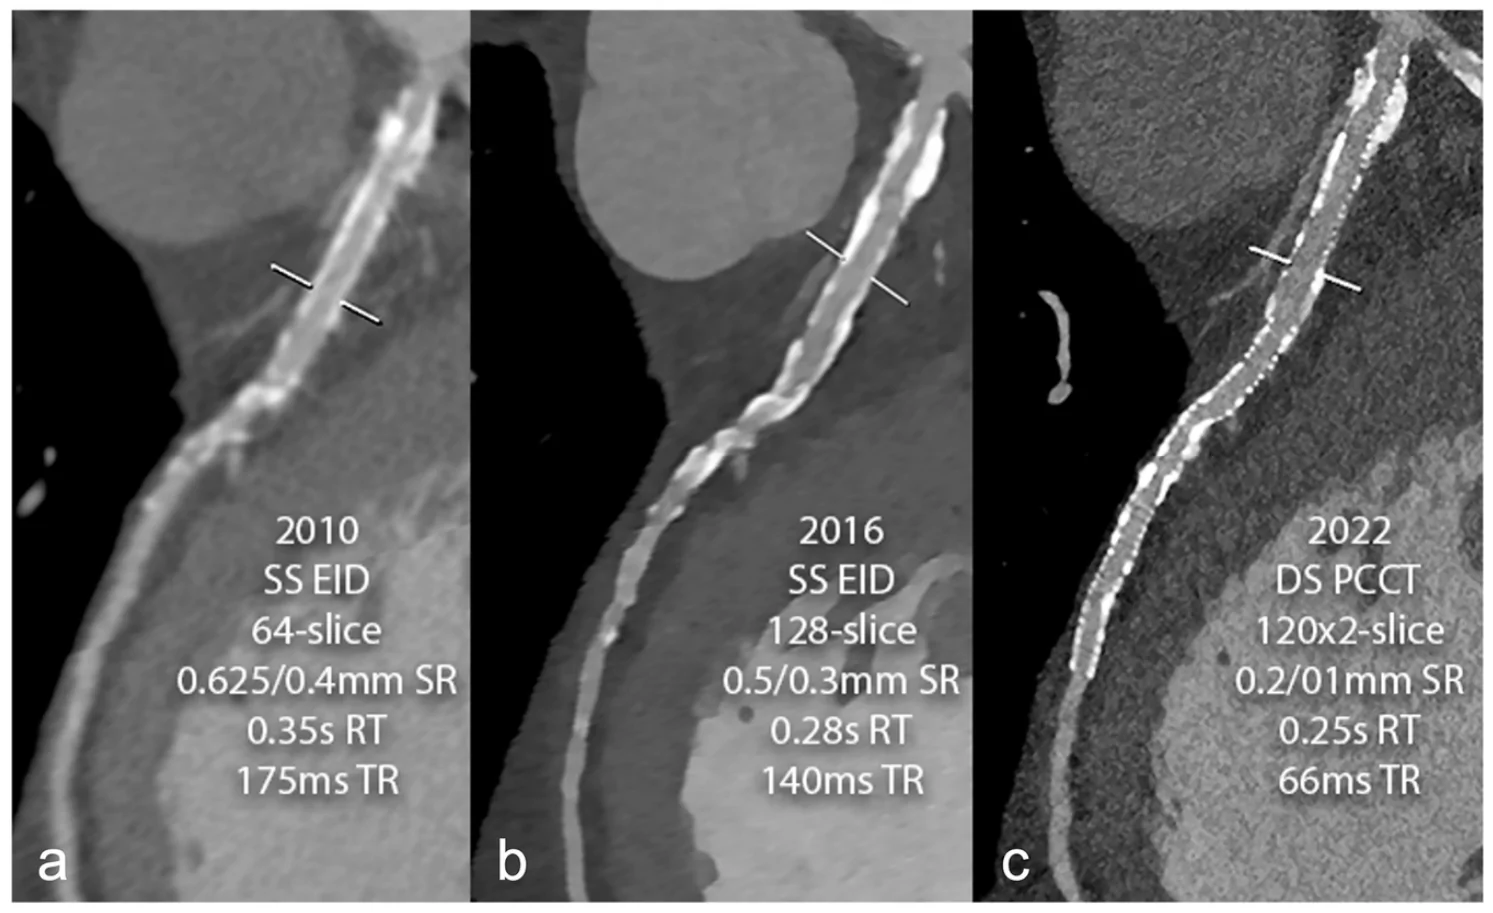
Clinical evolution of CCTA for coronary stent assessment over 15 years in the same approximately 70-year-old patient with coronary artery disease and multiple LAD stents. (a) 2010 single-source 64-slice CT (0.625-mm slices; 175-ms temporal resolution). (b) 2016 single-source 128-slice CT (0.5 mm; 140 ms). (c) 2022 dual-source photon-counting CT (0.2 mm; 66 ms). Progressive improvements in image sharpness and reductions in motion and blooming artifacts from stents and calcifications are evident. CCTA, cardiac CT angiography; LAD, left anterior descending artery; PCCT, photon-counting CT; SS, single source; DS, dual source [59].
